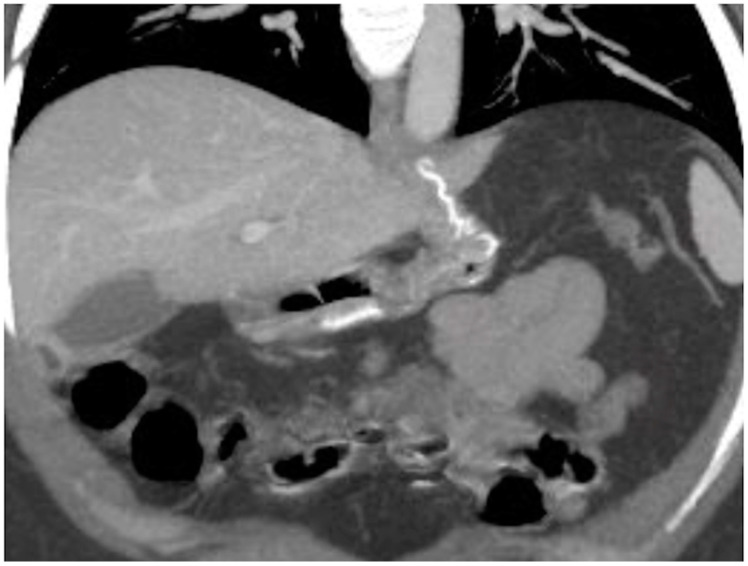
Surgical suture of sleeve gastrectomy seen on CT coronal oblique CT view.
